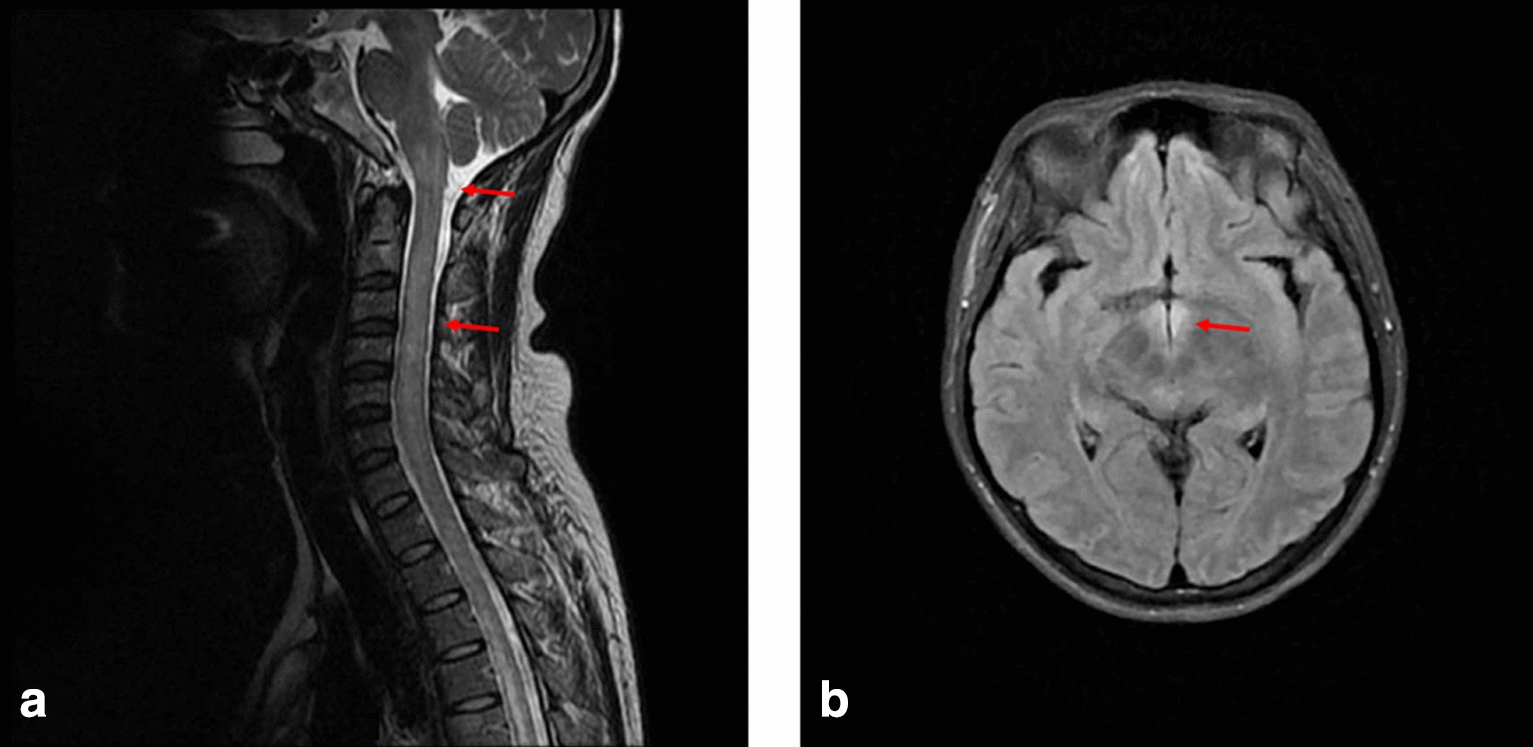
MRI T2 sequence of case 1 showing hyperintense in the medulla oblongata, cervical medulla, and thoracic medulla (highlighted by arrows) in the a sagittal planes, and hyperintense in the bilateral hypothalamus in the b transverse planes
